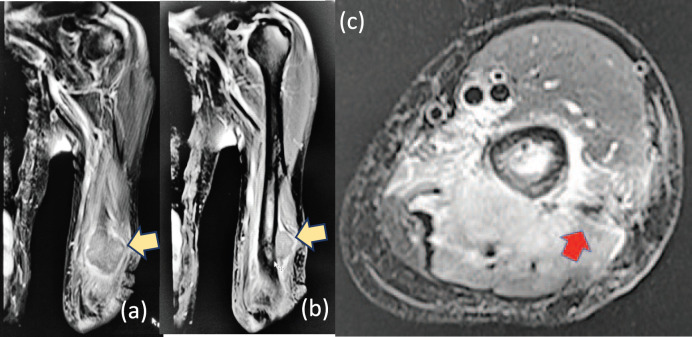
T2-weighted (a): coronal, (b): sagittal and (c): axial MRI confirming a heterogeneously enhancing intramuscular mass involving the triceps (yellow arrows), measuring 3 cm × 4 cm × 4.5 cm associated with cortical erosion of posterolateral humerus and partial tumoural encasement of the radial nerve (red arrow).
